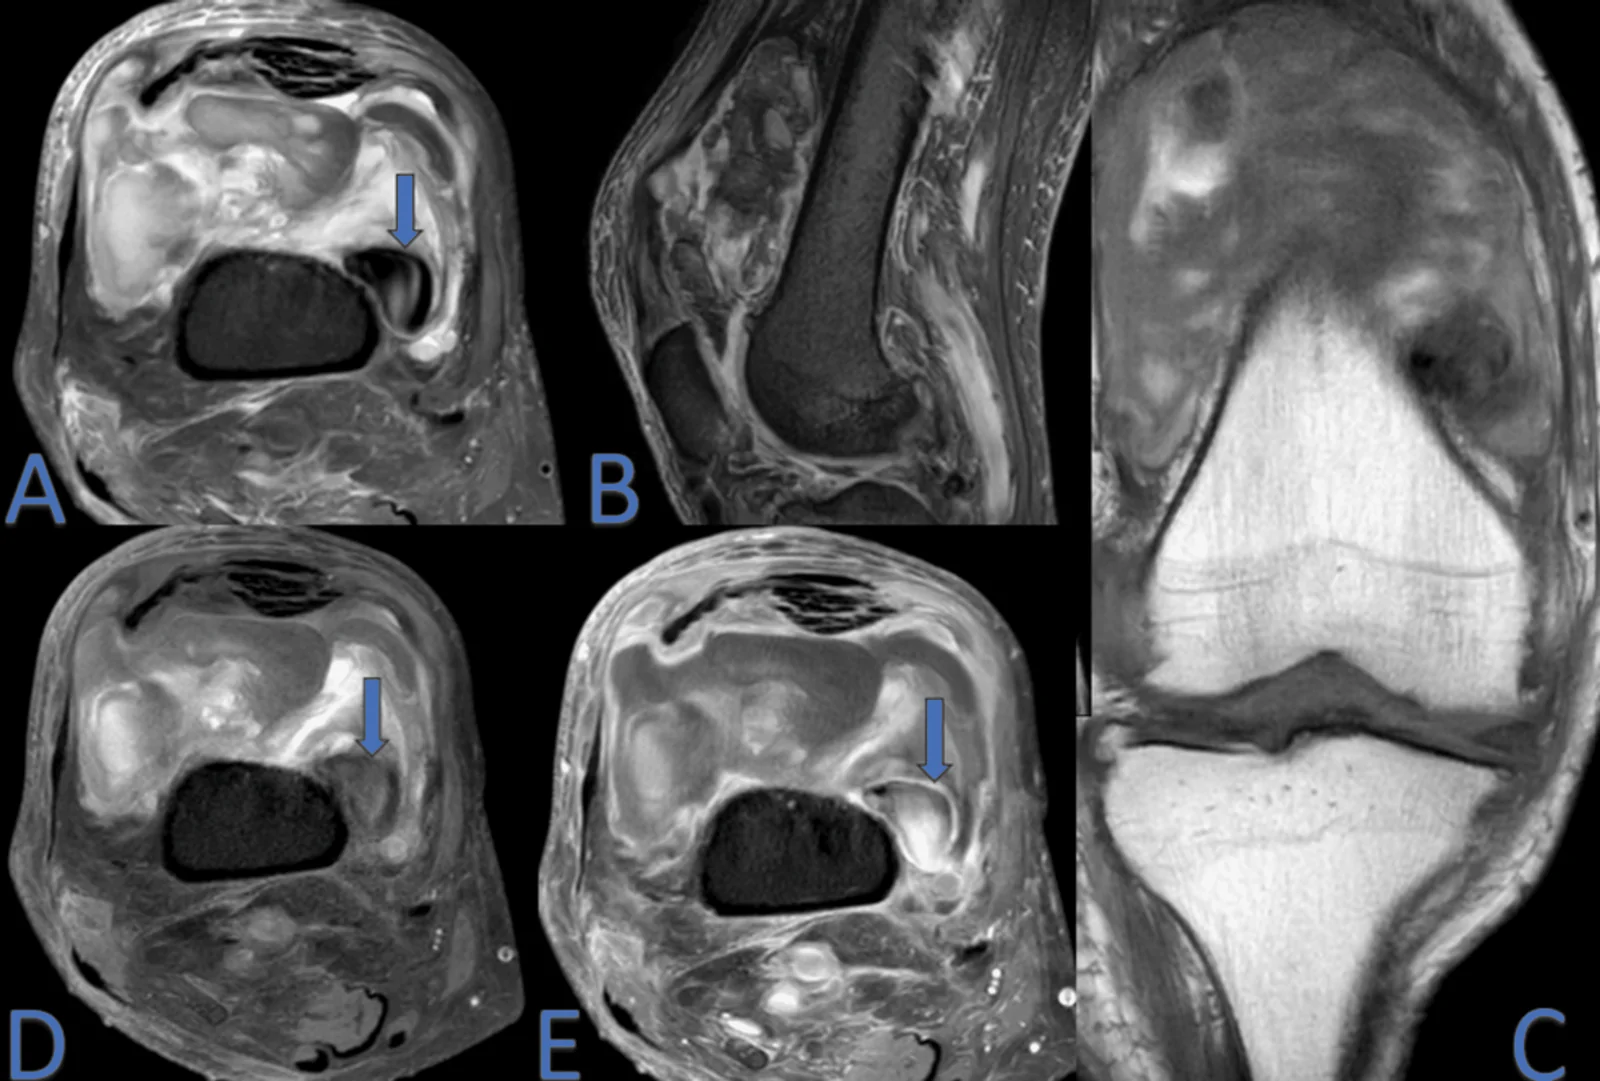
MRI images of the knee (A) Axial PD SPAIR, (B) Sagittal GRE, (C) Coronal T1, (D) Axial T1-weighted FS Pre-contrast, (E) Axial T1-weighted FS Post-contrast of the knee demonstrating large complex knee joint effusion, with high T1 signal and heterogeneous signal on fluid-sensitive sequences and extensive blooming artifact. Post-contrast heterogeneous enhancement is noted with synovitis. Arrow representing a small focal area of signal void with partial enhancement noted along the deep aspect of the suprapatellar region, demonstrating features consistent with pulsation artifact from an intra-articular vessel.
